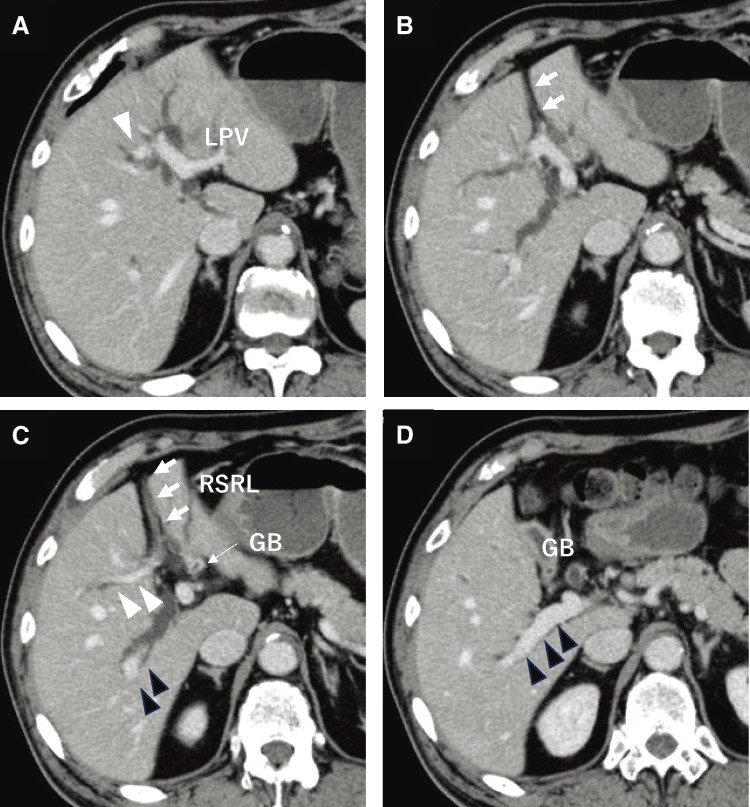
Contrast-enhanced computed tomographic images of the patient. The posterior branch of the PV (black arrowheads) is ramified independently (Ppost-i type, D), followed by the ramification of the anterior branch of the PV (white arrowheads) and the LPV (A–C). The ant-PV formed a right-sided umbilical portion of the PV and was connected to the round ligament (white arrows) (B, C). The RSRL (white arrows) was located to the right of the gallbladder (C, D). ant-PV, anterior branch of the portal vein; GB, gallbladder; LPV, left portal vein; PV, portal vein; RSRL, right-sided round ligament
